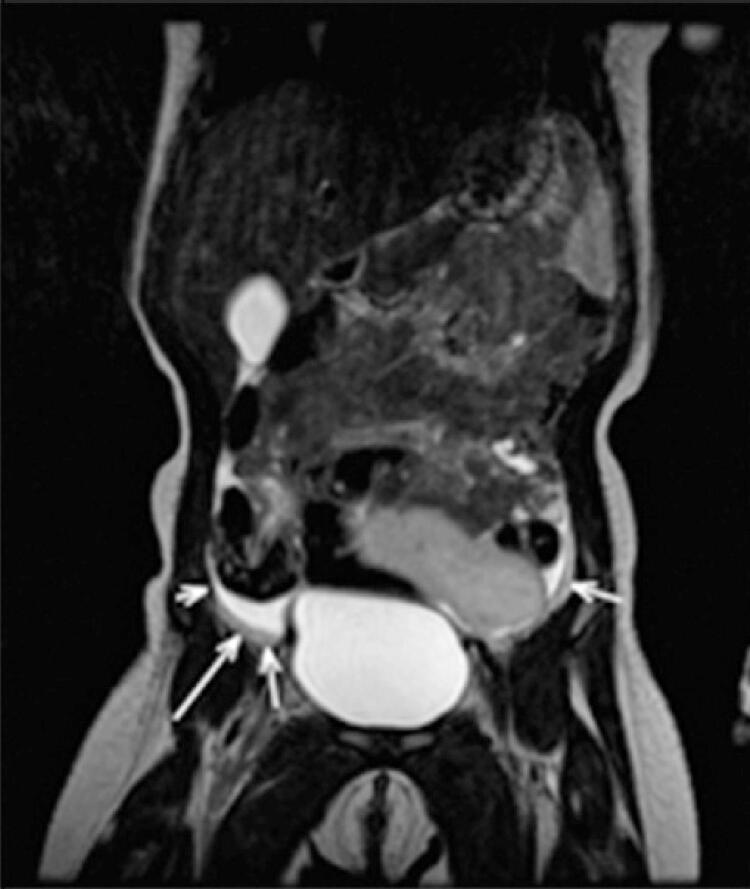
Coronal T2 magnetic resonance imaging sequence without gadolinium demonstrates small amount of free fluid in the pelvic cavity
